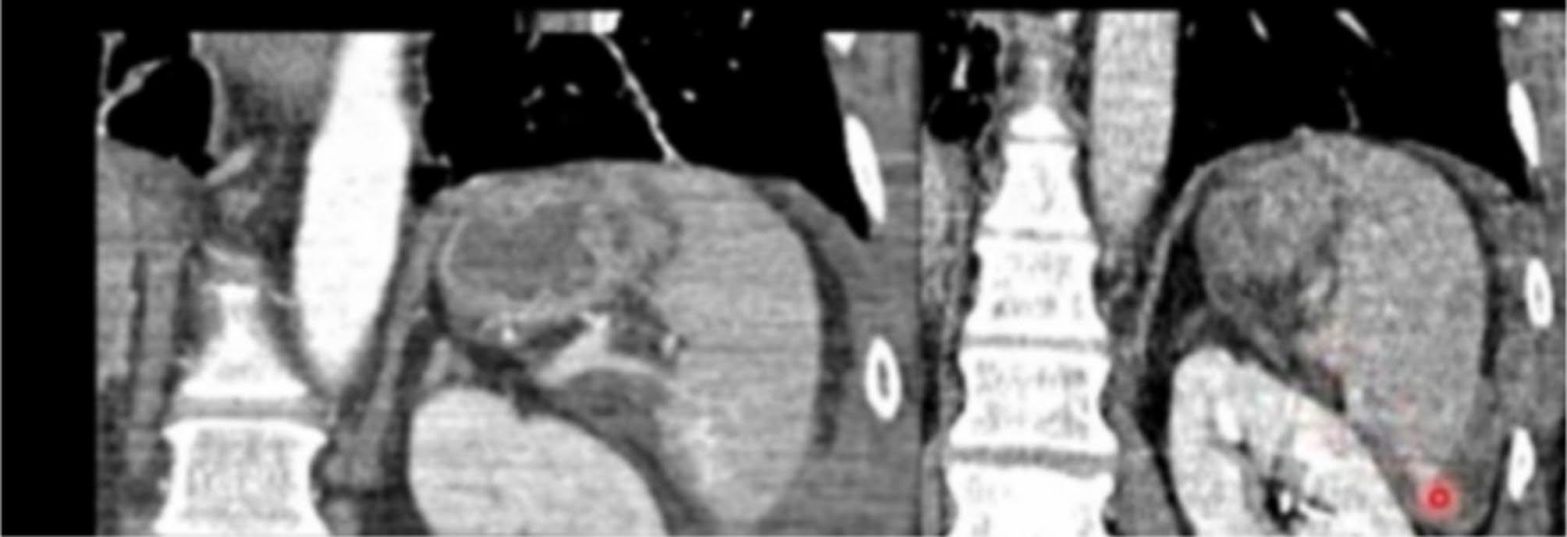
CT contrast showing grade 5 tear after falling from up
